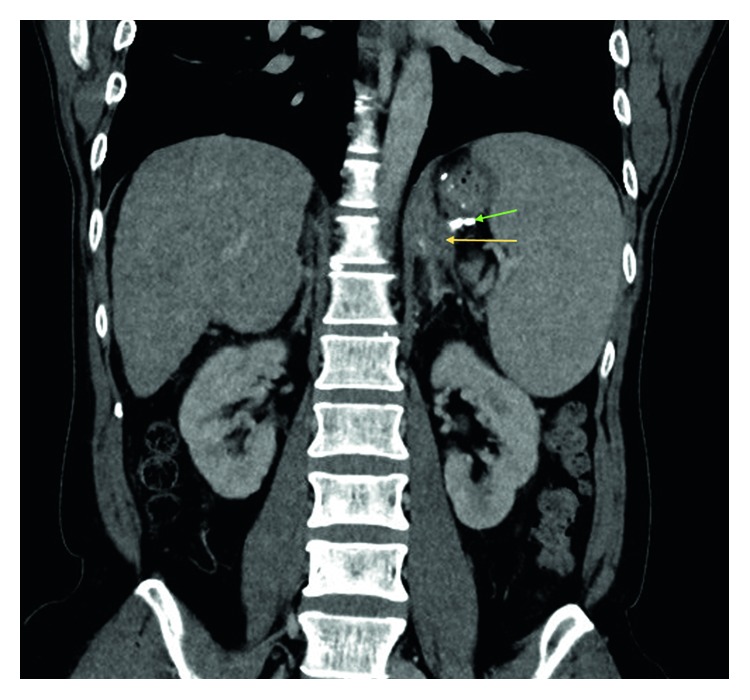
Computed tomography view of the abdomen (in coronal view) after completion of antibiotics. The previously seen retrogastric abscess has completely resolved leaving behind a small area of scarred tissue (yellow arrow). The residual N-butyl-2-cyanoacrylate in the perigastric region is still visible (green arrow).
